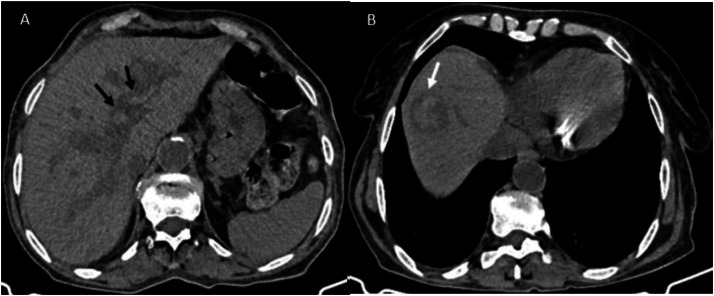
(A) Unenhanced computed tomography shows dilated intrahepatic bile ducts with hyperdense heterogeneous content suggestive of haemobilia (black arrow). (B) It also demonstrates a biloma with hemorrhagic components (white arrow).
